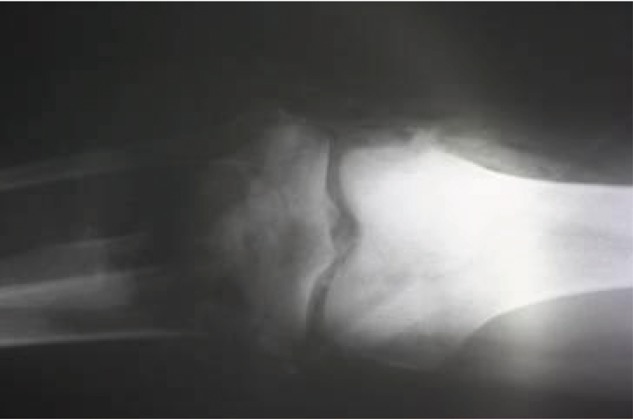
X-ray of the patient at the time of admission.
